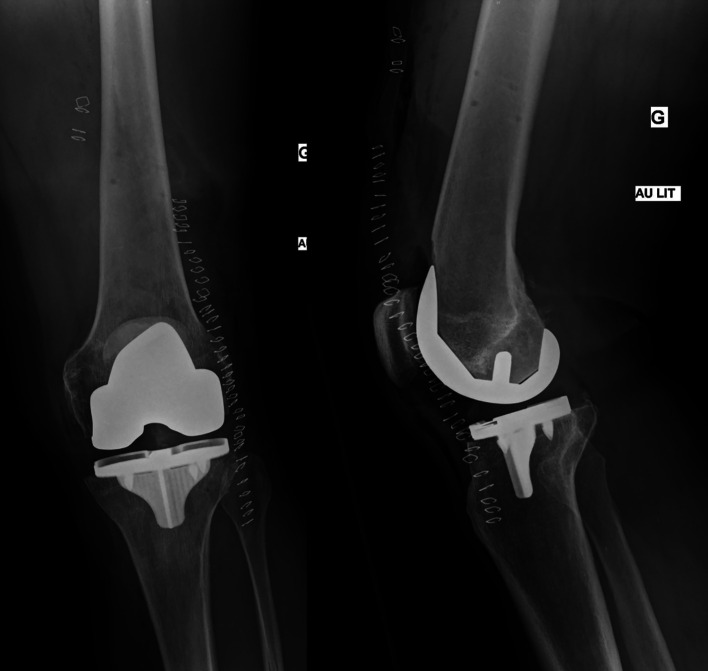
Postoperative X-rays after the placement of the final implant with a cementless femoral and tibial component (Triathlon, Stryker, MI, USA).
